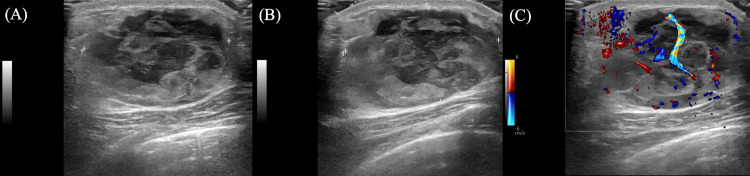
Targeted breast ultrasound of the right breast A. Grayscale ultrasound image, radial view. B. Grayscale ultrasound image, anti-radial view. C. Color Doppler image, radial view. Targeted ultrasound reveals an oval, parallel-oriented, circumscribed mass with heterogeneous echotexture, internal vascularity, and posterior acoustic enhancement, measuring 4.2 × 3.4 × 3.9 cm. The lesion is located at the 1:00 position, approximately 5 cm from the nipple.
